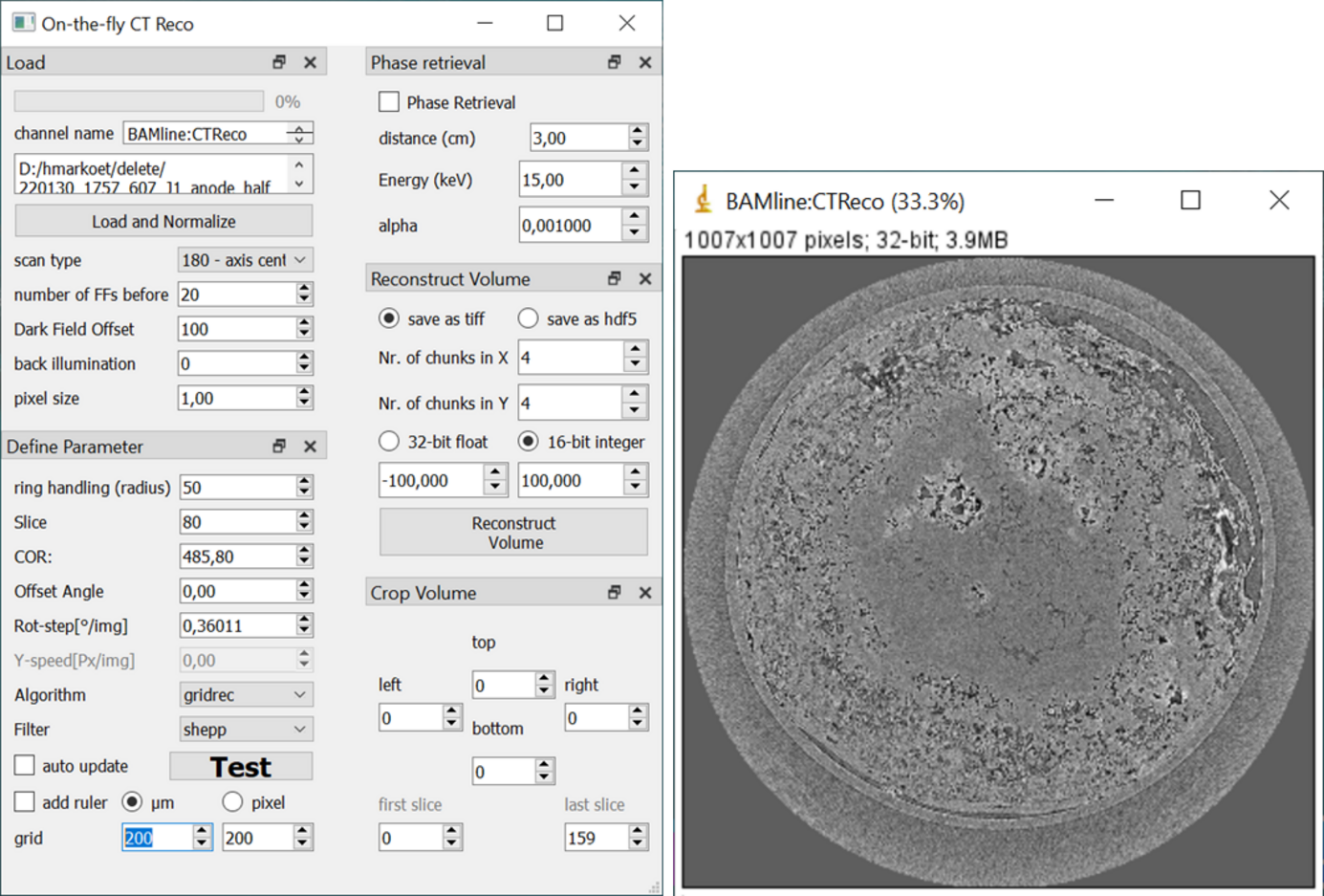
Left: graphical user interface for *On-the-fly CT reconstruction*. Right: the parameters are tested on one slice, which is sent to *ImageJ*.
